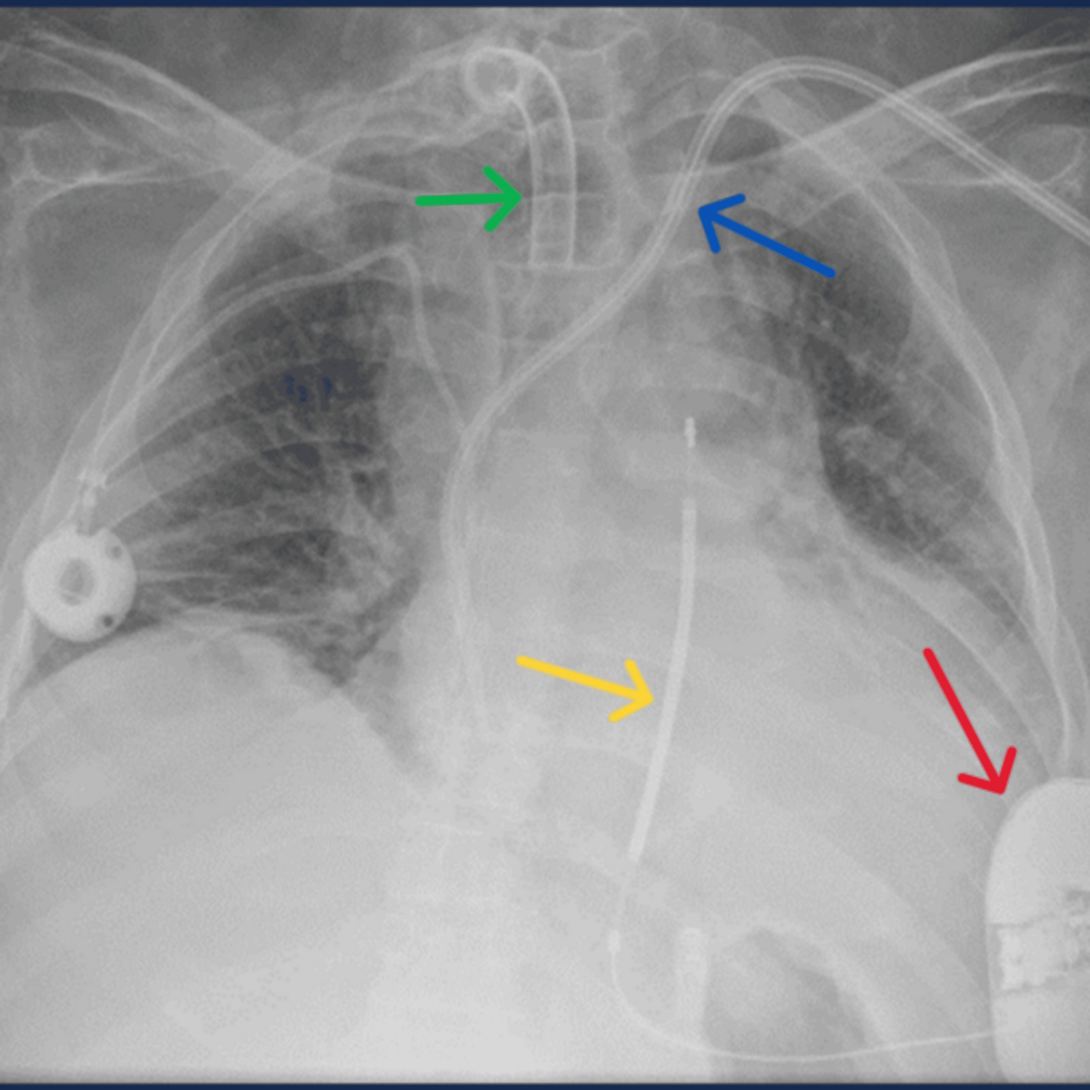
A chest radiograph obtained following the implantation of a subcutaneous implantable cardioverter defibrillator (S-ICD). Red arrow: S-ICD pulse generator box; yellow arrow: S-ICD electrode; blue arrow: left internal jugular tunneled central venous catheter; green arrow: port-a-cath in the right subclavian vein for long-term venous access
